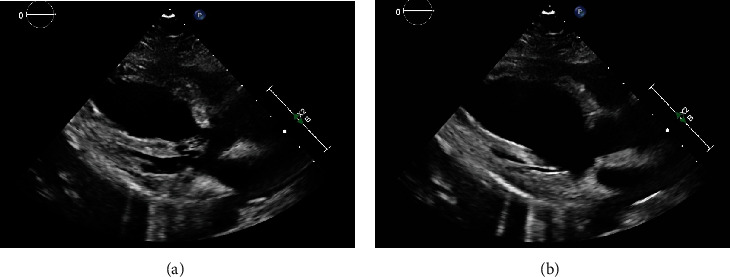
(a) Systole and (b) diastole: transthoracic echocardiogram: left ventricular ejection fraction of 25-30% with reduced systolic function. Akinesis of the basal-mid anteroseptal wall and hypokinesis of the entire inferior wall.
